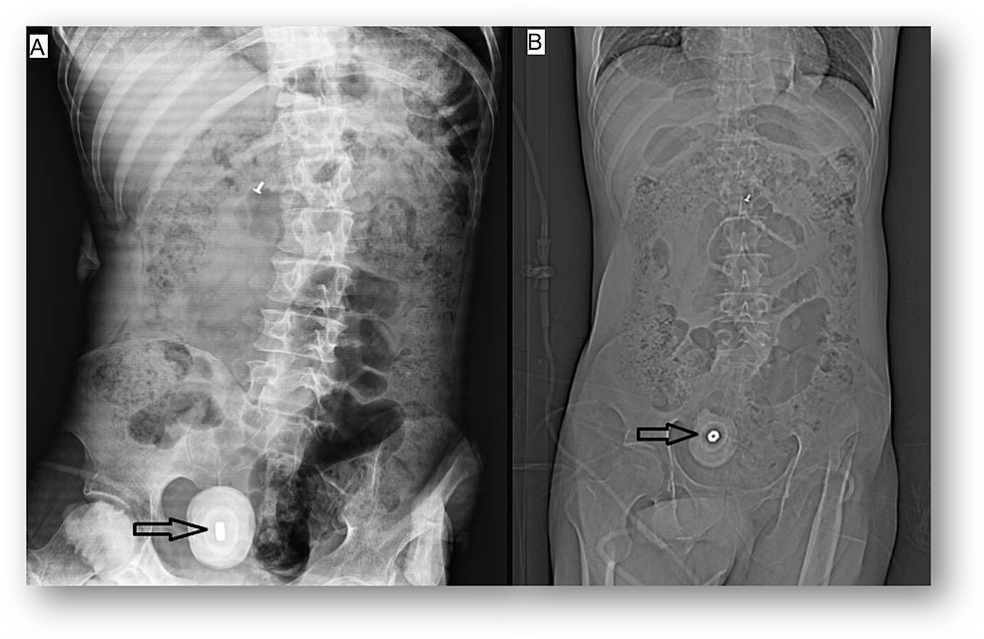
(A) Abdomen Rx with a radiopaque oval lesion on the pelvis (black arrow). (B) CT of the abdomen scout with an oval lesion with metallic density on its center (black arrow). Rx: radiography; CT: computed tomography
